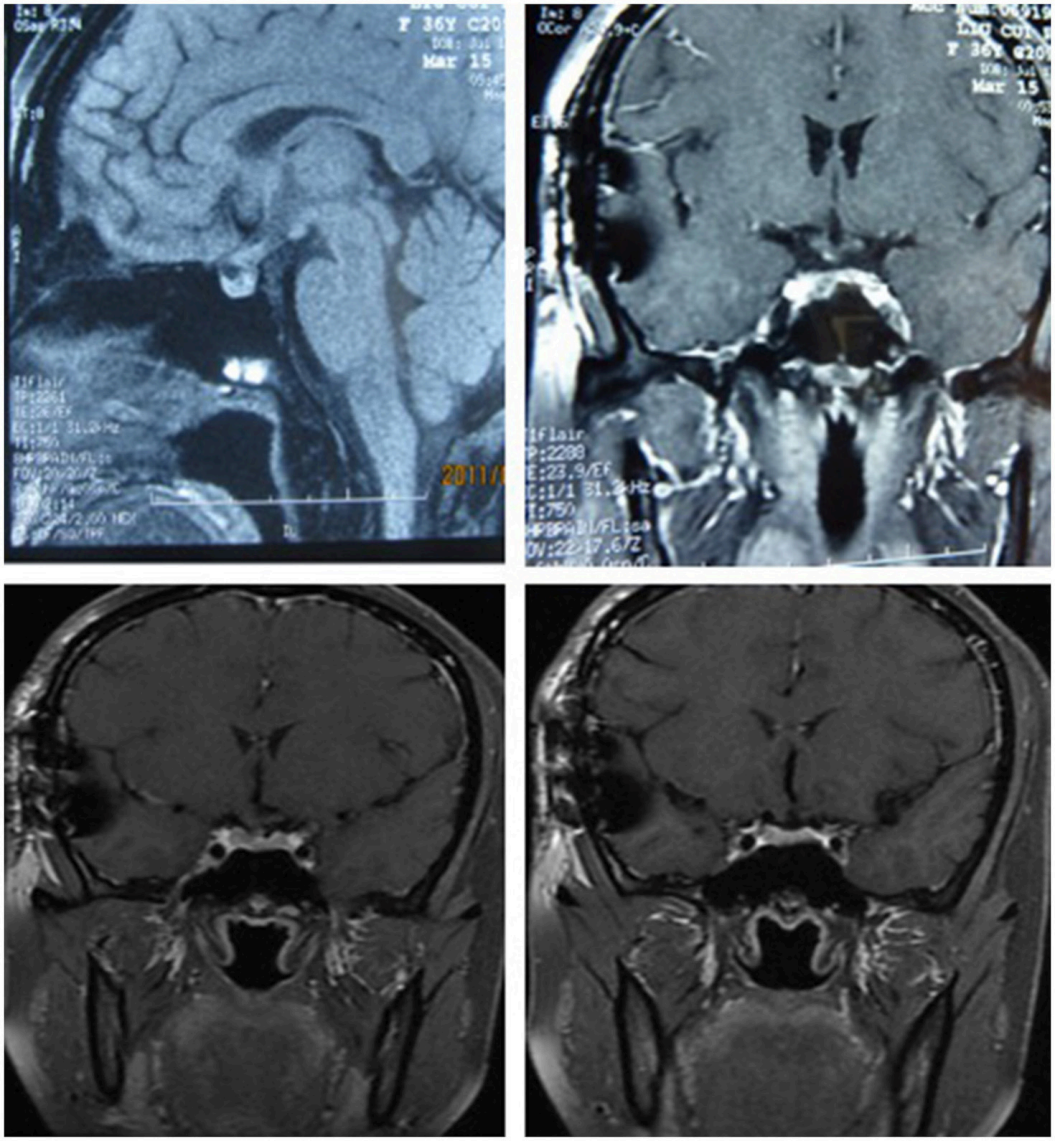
The MRI of cranial and pituitary (1 year after surgery). No expansion of sella region, no signs of local tumor, no abnormal enhancement, clear boundary of optic chiasm, no signs of tumor recurrence.
